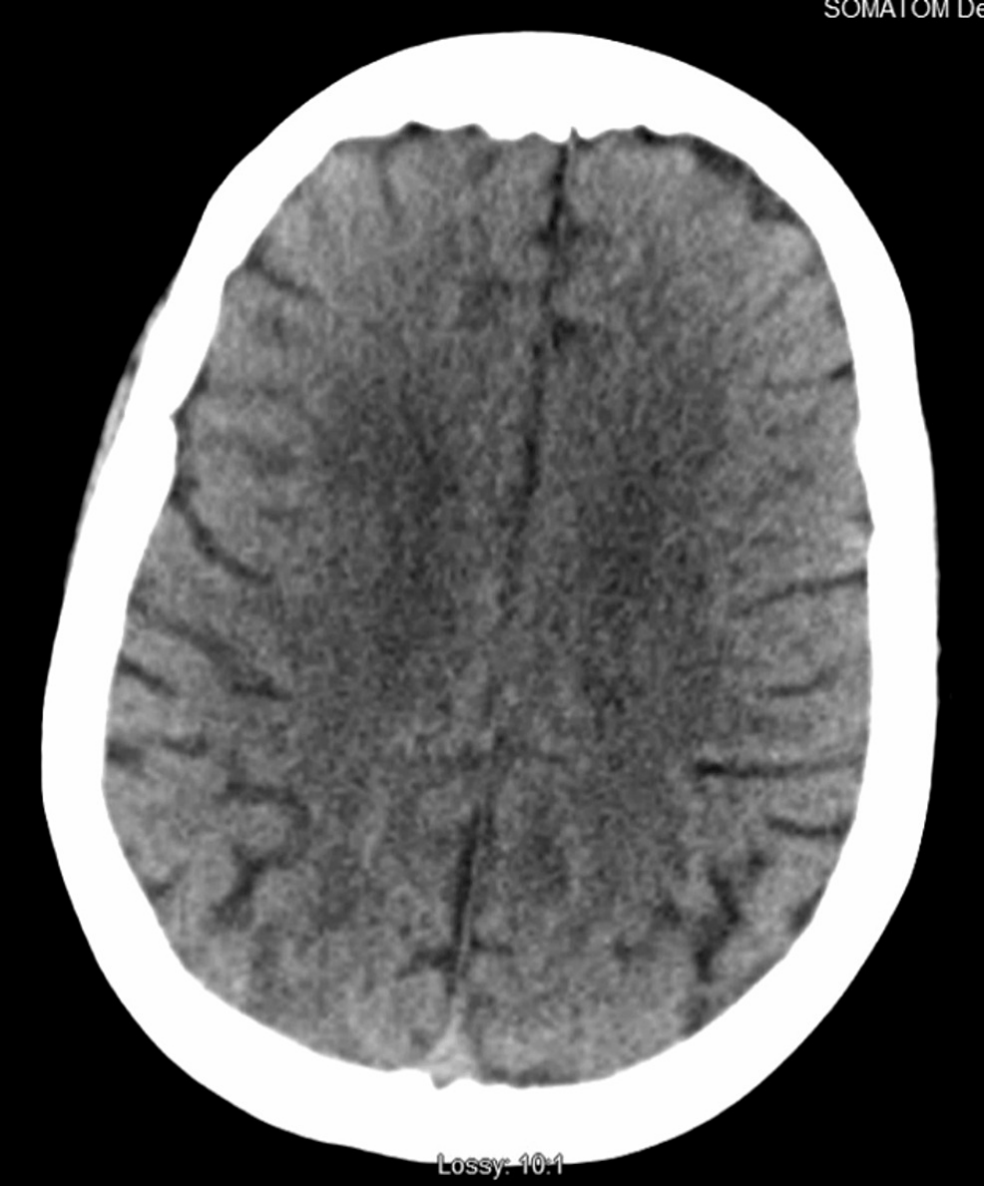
CT scan of the head 24 hours later after treatment with IV corticosteroids. Significantly improved gray-white matter differentiation. No acute intracranial abnormality is noted.
